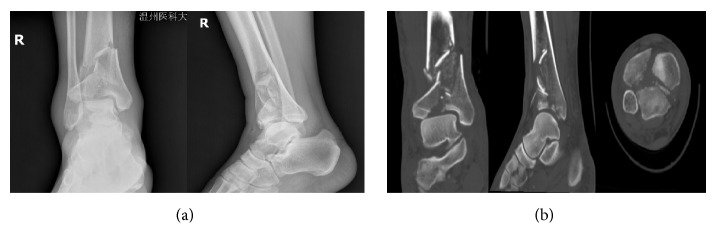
The patient's preoperative radiological characteristics of Pilon fracture. (a) The anteroposterior and lateral X-ray. (b) CT images of the fracture.
